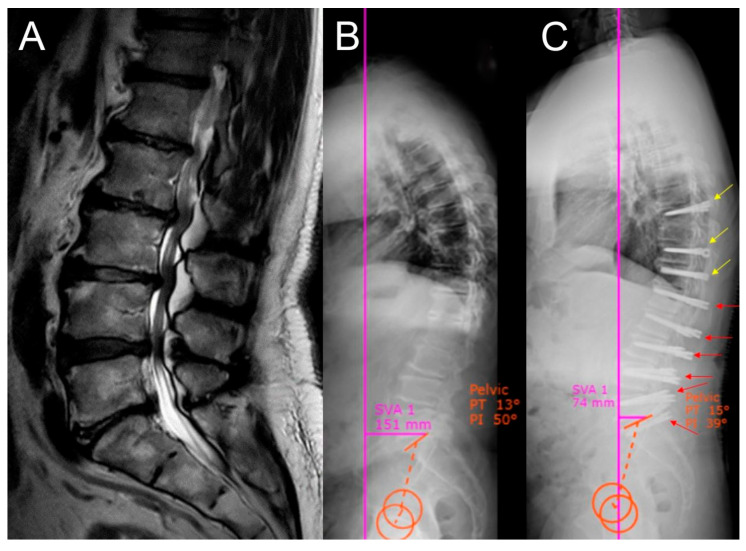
Radiological images of a patient presenting with walking difficulty and low back pain. Decompression and dynamic stabilization using Dynesys were performed. (A) Preoperative sagittal MRI image showing Pfirrmann grade 4 intervertebral disc degeneration. (B) Preoperative lateral X-ray image showing sagittal imbalance and pelvic parameters. (C) Postoperative lateral X-ray image showing improvement in sagittal balance.
